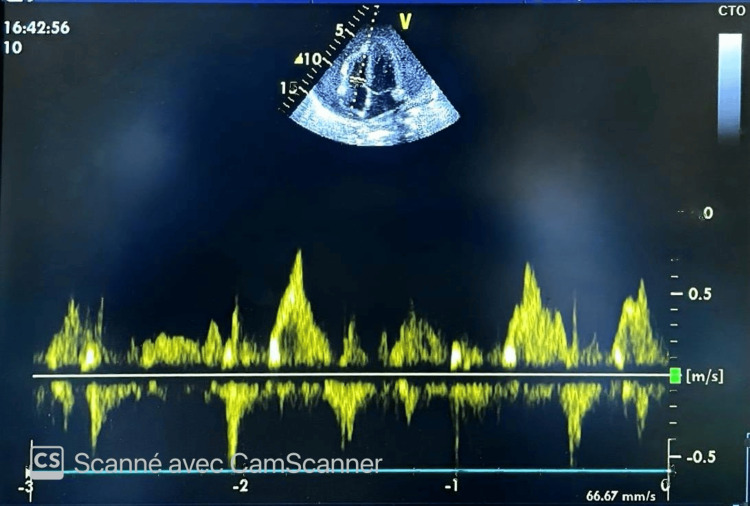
Tricuspid inflow variations. Pulsed-wave Doppler of the tricuspid inflow demonstrating marked (>50%) respiratory variation. This finding, in conjunction with right-sided chamber collapse, is highly suggestive of tamponade physiology.
